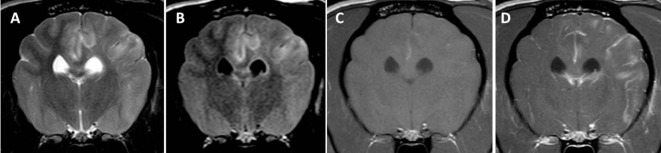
Transverse magnetic resonance images (3 T) of a Pug (3 years, male) with necrotizing meningoencephalitis. Diffuse T2 (A) and fluid-attenuated inversion recovery (B) hyperintensities predominantly on the left in the prosencephalon resulting in the complete loss of cortical gray and white matter distinction. There is a patchy parenchymal and a leptomeningeal enhancement after a contrast injection [(C) T1-weighted native; (D) T1 weighted after contrast injection]. The midline is slightly shifted to the right side caused by the expansion of the prosencephalon on the left-hand side.
